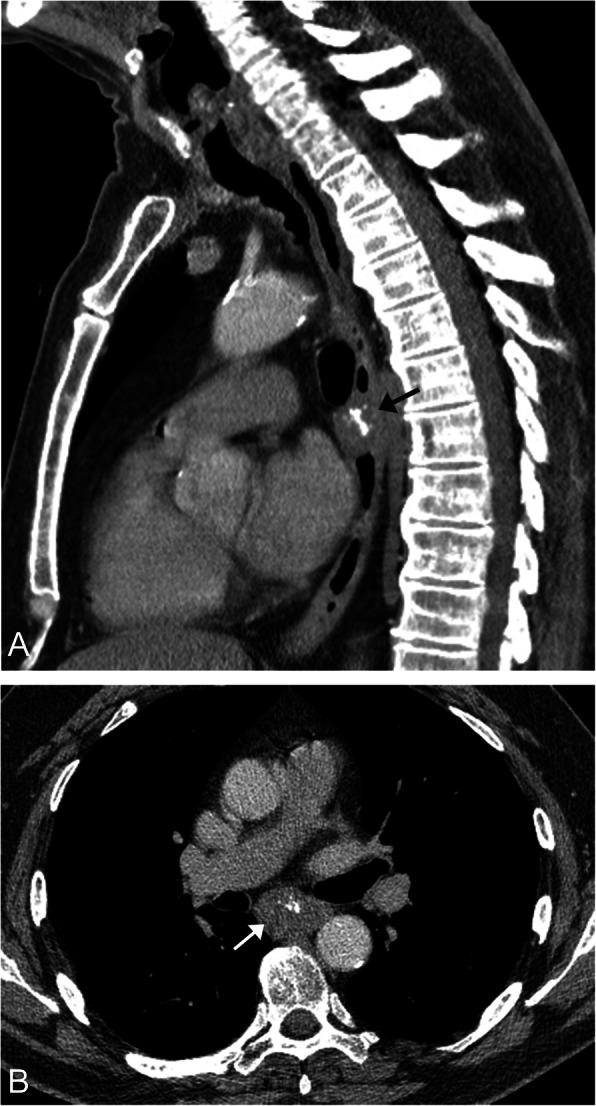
Leiomyoma. Sagittal (a) and axial (b) CT image of the chest demonstrates an ovoid hypoattenuating mass located in the mid esophageal wall (arrow). The presence of calcifications within this mass is highly suggestive of a leiomyoma
